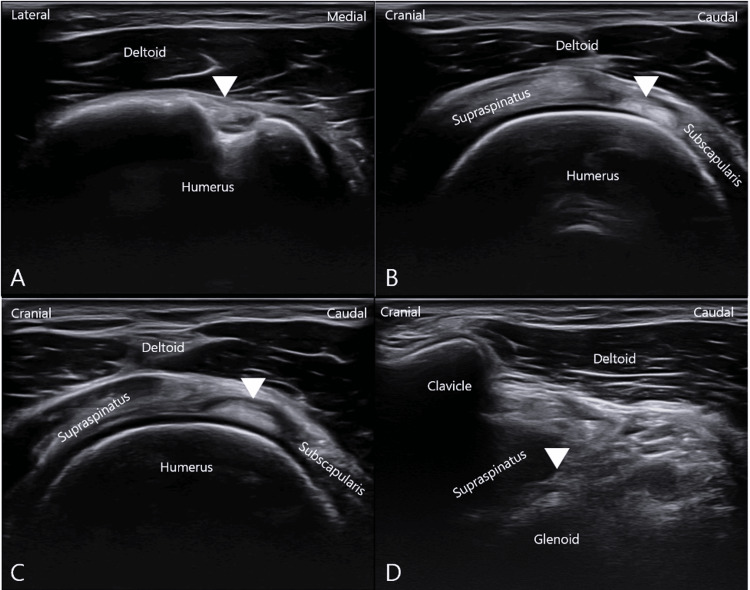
Stepwise short-axis ultrasound tracking of the long head of the biceps tendon to the superior labral-biceps anchor complex in a healthy volunteer Representative static short-axis ultrasound images of the structured biceps-tracking sequence. (A) LHBT is identified within the bicipital groove. (B) Proximal tracking through the rotator interval region. (C) Visualization of the LHBT entering its intra-articular course. (D) Final confirmation of the biceps anchor at the supraglenoid tubercle/superior labral attachment Image courtesy of Dr. Yonghyun Yoon
